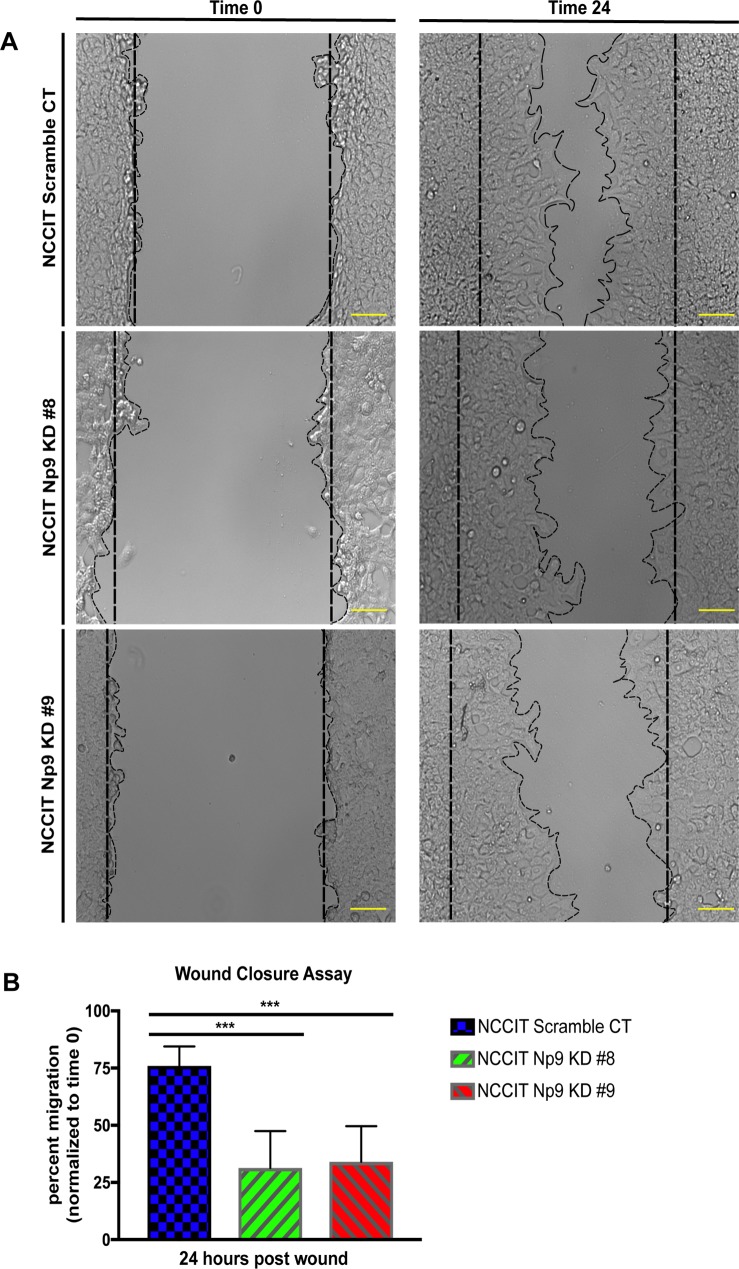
Np9 is essential for cell migration and wound closure. (A) NCCIT scramble control and NCCIT Np9 KD clones 8 and 9 were used for the migration assay. A total of 200,000 cells for each cell type were transferred into a 12-well plate. The following day, a scratch was created down the middle, and pictures were taken at time 0 and at 24 hours. The images are representative of 3 independent experiments done in duplicate. Scales bars, 100 μm. (B) The wound was measured at time 0 and at 24 hours. The percent of migration was plotted over time 0. The measurements are representative of 3 independent experiments done in duplicate. The width of the wounds was measured in each image and totaled for scramble CT (n = 26), KD #8 (n = 27), and KD #9 (n = 28). The reduction in the expression of Np9 in NCCIT cells resulted in reduced cell migration post-wound as compared to scramble control cells. T bars denote the standard deviations of the means; P values were determined using student t-test analysis. P*<0.0001.
